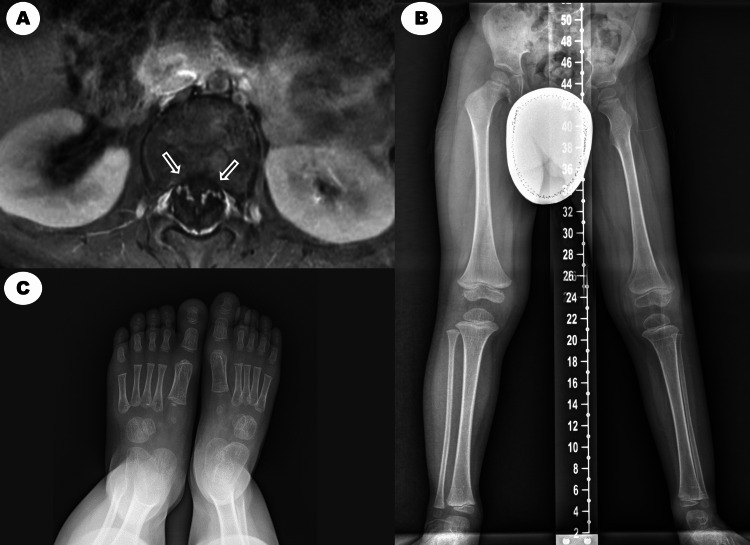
Spinal MRI and Follow-up Radiographs Demonstrating Chronic Left Lower Extremity Atrophy (A) Spinal MRI obtained one month after symptom onset demonstrates prominent enhancement of the bilateral ventral nerve roots. Arrows indicate the enhancing ventral nerve roots. (B) Radiograph obtained two years later shows diffuse atrophy of the left lower extremity extending from the hip to the foot. (C) A close-up radiograph of the left foot demonstrates distal atrophy compared with the contralateral side, consistent with chronic neurogenic atrophy.
